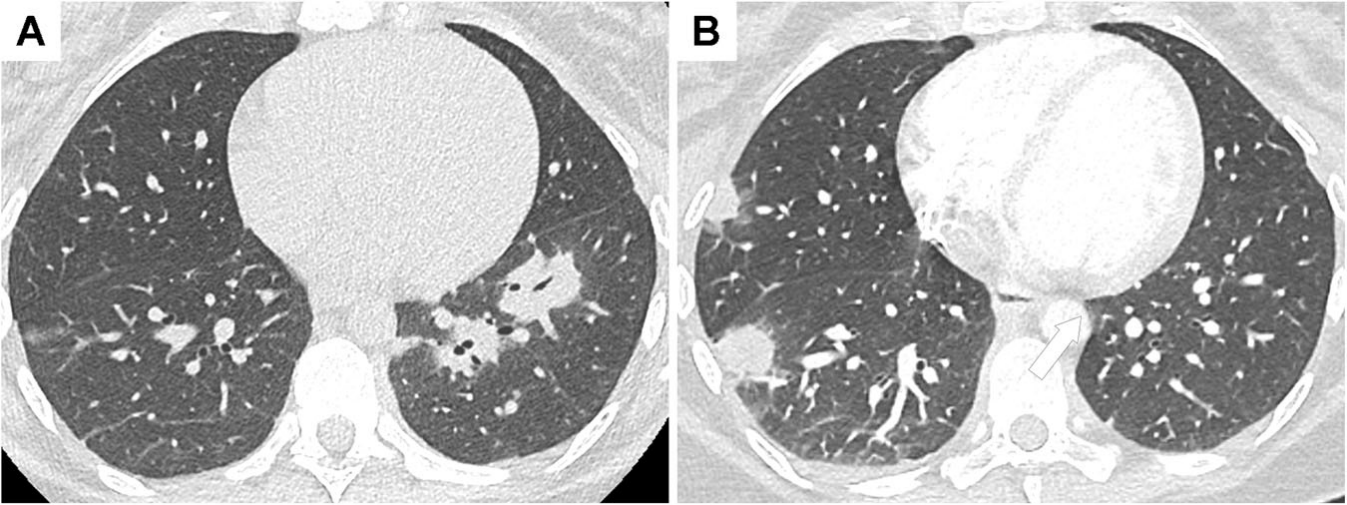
Recurrent organizing pneumonia in a 28-year-old patient with Crohn’s disease. A CT image in the axial plane shows peribronchovascular consolidations in the left lower lobe consistent with organizing pneumonia. These lesions disappeared with corticosteroids. B Few months after corticosteroids withdrawal, a recurrence of organizing pneumonia was observed in other lung areas
